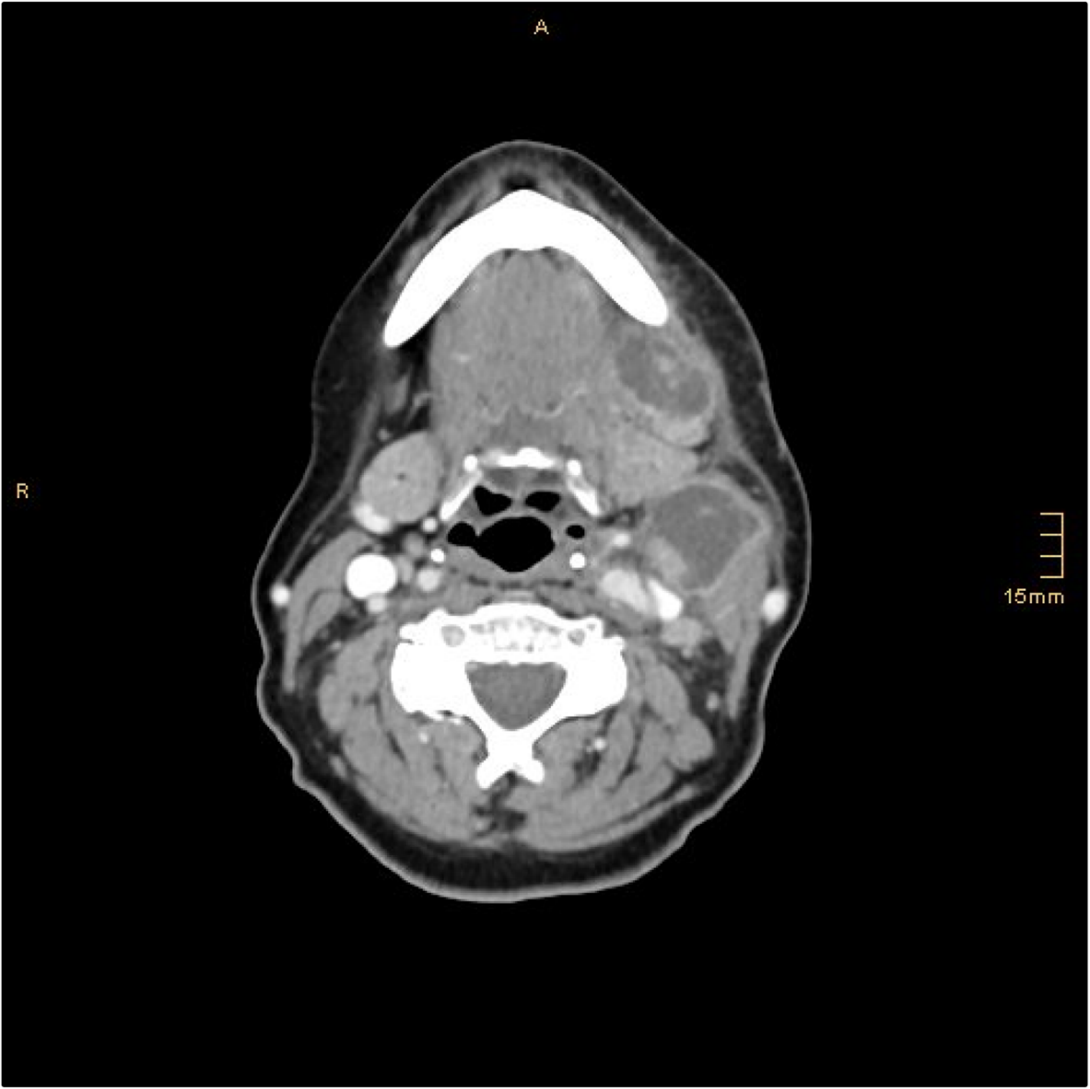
Computed tomography image of the abscess formations in the left submandibular region
